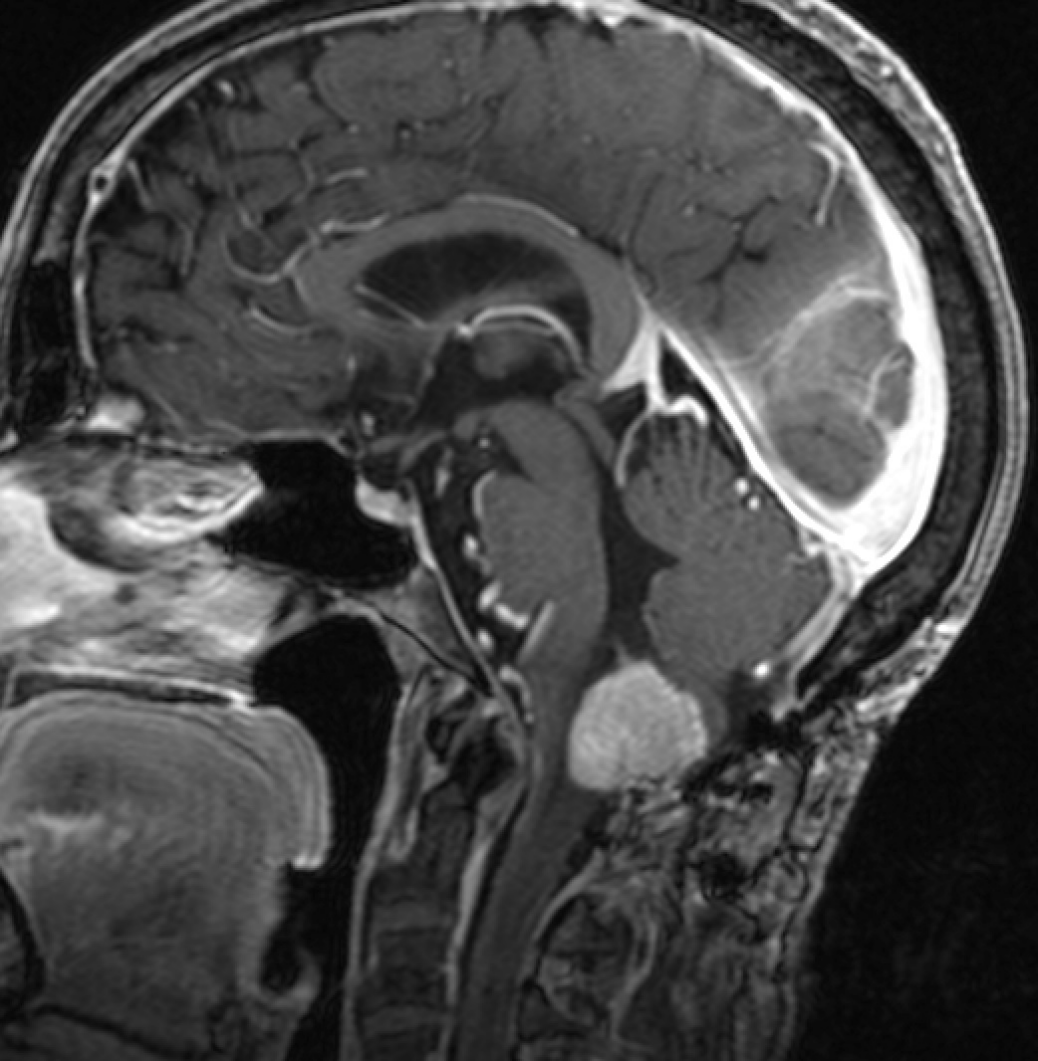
Recurrent Hemangioblastoma After Initial Resection MRI of the brain with contrast revealing a hemangioblastoma at the cervicomedullary junction.
